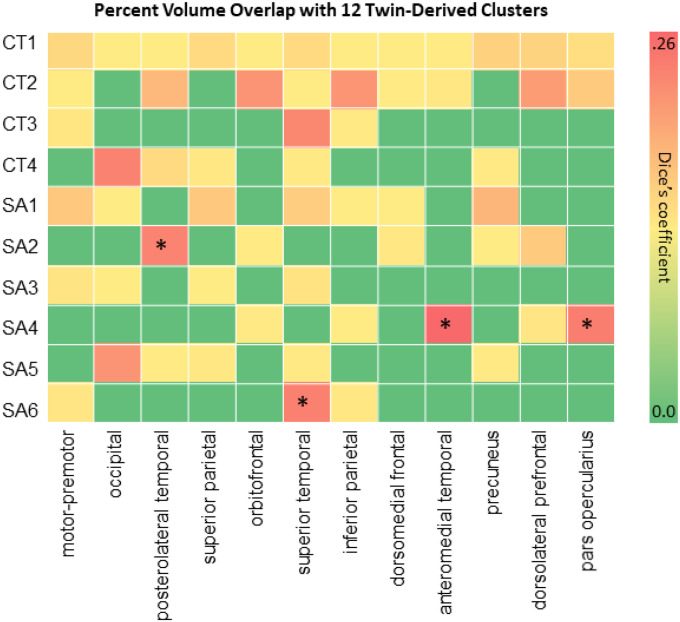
Genetic Parcellation of Twin Brains (A) We examined the percent volume overlap as measured by Dice’s coefficients for the overlap between 6 SA GIBNs and the 12 clusters reported by Chen et al. (2012) derived from twin brain data. *FDR-corrected p-values for percent volume overlap with 12 clusters are indicated by an asterisk. Abbreviations: SA=surface area, CT=cortical thickness, GIBN=genetically informed brain network.
